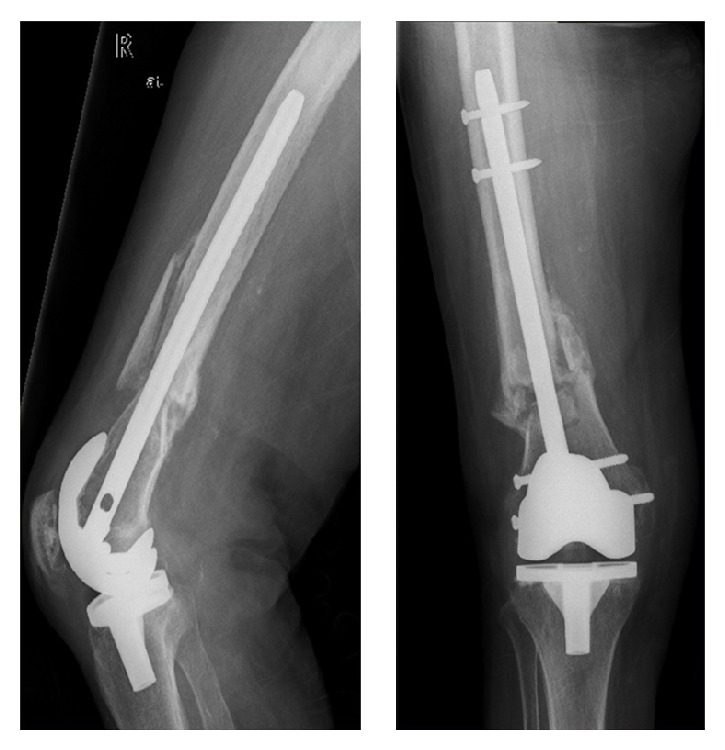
Five months of postoperative AP and lateral right knee X-rays showing signs of bone healing.
